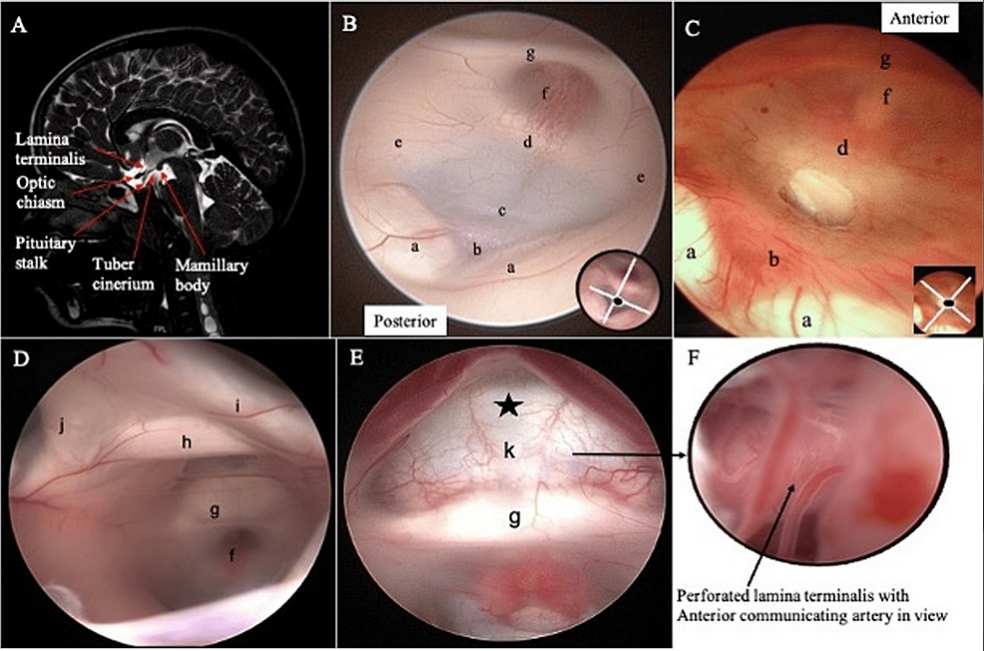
Anatomical images of the floor and anterior part of the third ventricle. (A) Mid-sagittal CSF DRIVE MRI brain shows the anatomical structure at the region of the third ventricle. (B) In a normal ventricle, the floor of the third ventricle is whitish. (C) However, in a child with ventriculitis, the floor appears thick and reddish, and the inflammatory reaction makes it difficult to appreciate the adjacent anatomy (the image shows that the stoma is already created during the ETV procedure). (D) An angled neuroendoscope is used to view the anterior part of the third ventricle. (E) The lamina terminalis, a thin white matter membrane, appears intact. (F) In the case of perforated lamina terminalis, the anterior communicating artery can be seen. a, mamillary bodies; b, basilar artery underneath; c, the floor of the third ventricle; d, dorsum sella; e, hypothalamus; f, infundibular recess; g, elevation secondary to optic chiasm; h, anterior commissure; i, right fornix; j, left fornix; k and star, intact and thin lamina terminalis (can see the dura at the base).
